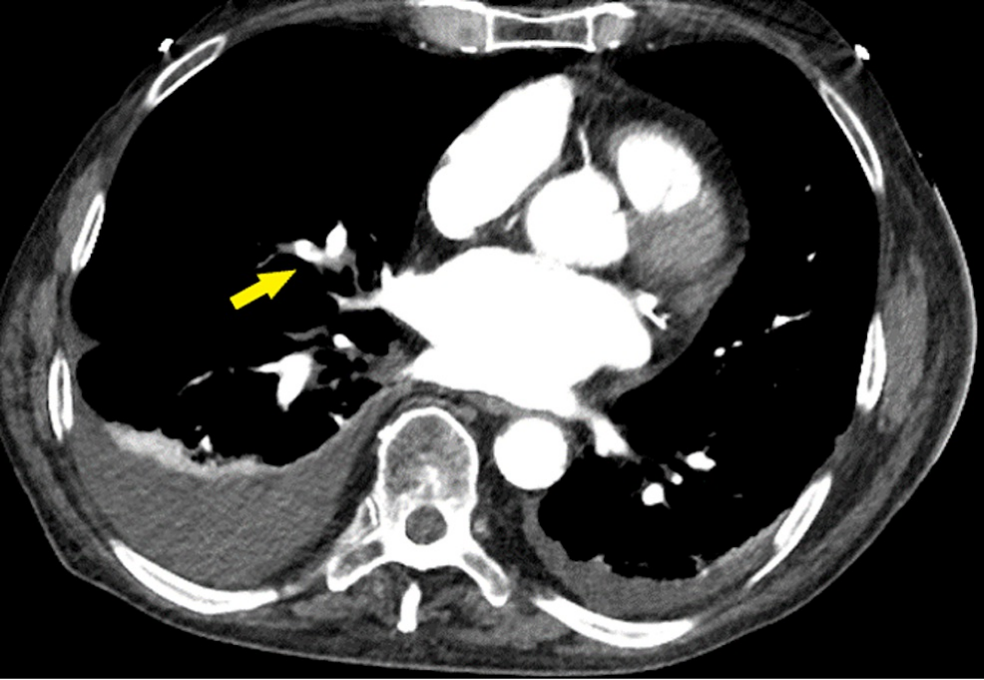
Computed tomography angiography showing pulmonary embolism, identified in the right middle lobe pulmonary arterial segments (yellow arrow).
